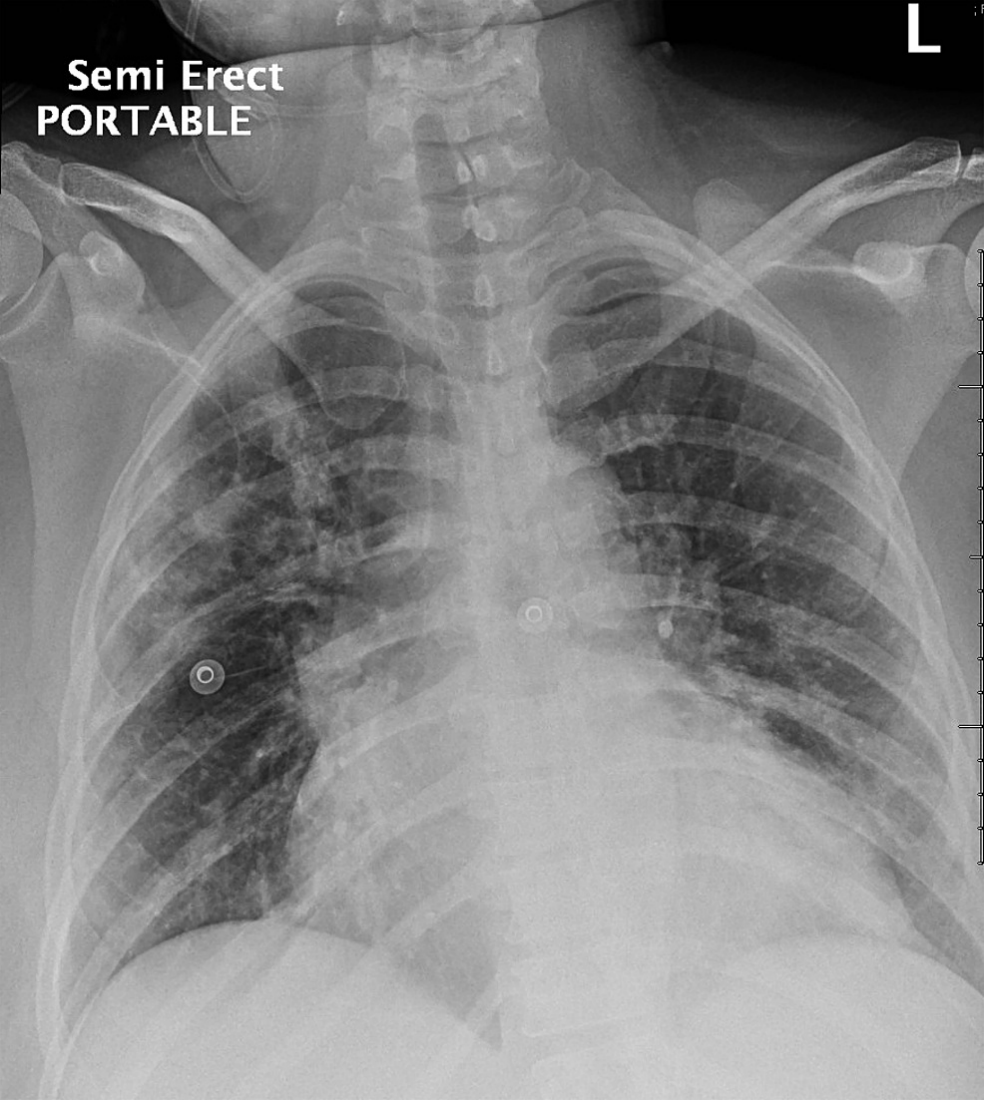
Chest X-ray of the patient Chest X-ray revealed patchy bilateral peripheral lung infiltrate. No pleural effusion pneumothorax is seen
